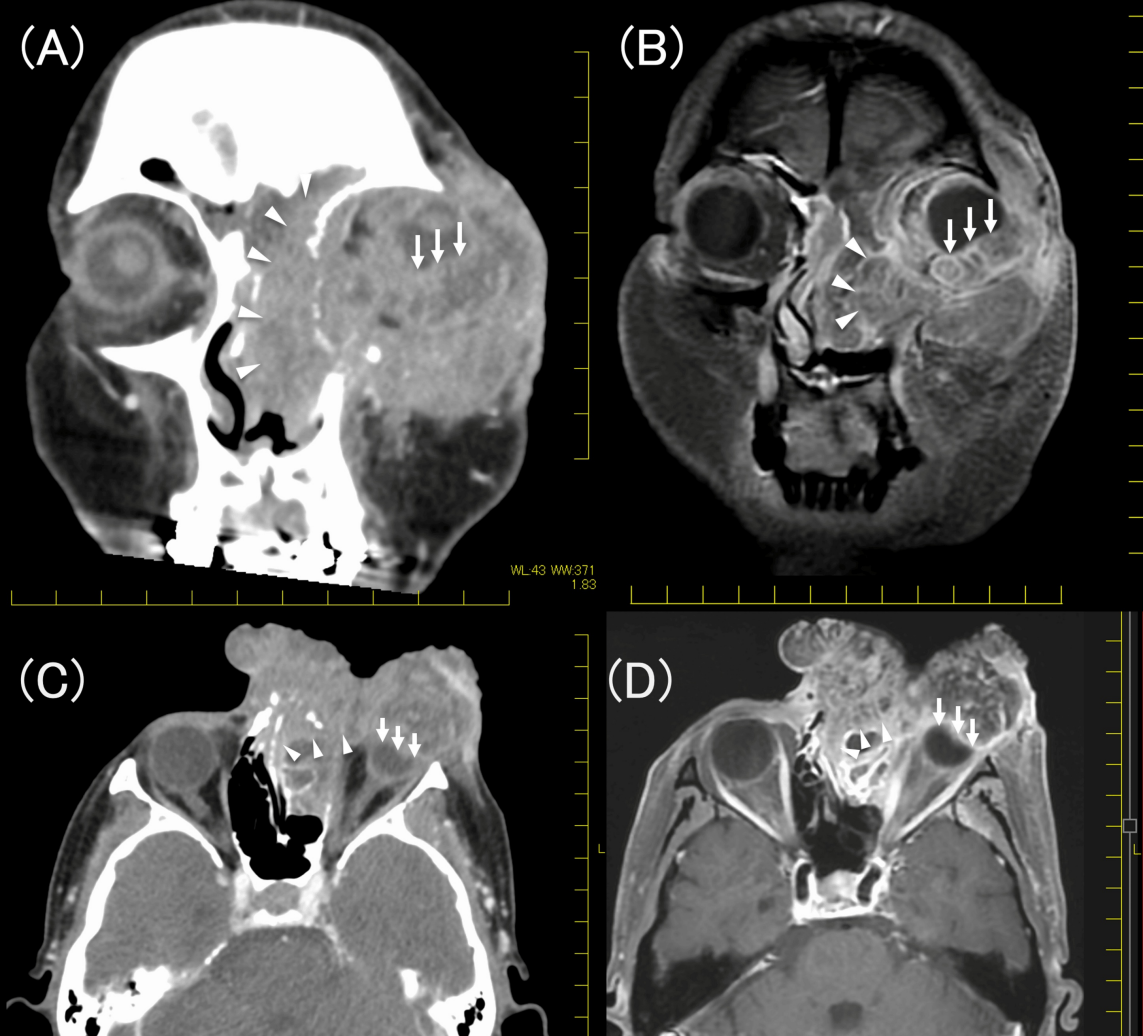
Computed tomography and magnetic resonance images. (A) Coronal contrast-enhanced computed tomography image. (B) Coronal T1-weighted contrast-enhanced magnetic resonance image. (C) Axial contrast-enhanced computed tomography image. (D) Axial T1-weighted contrast-enhanced magnetic resonance image The tumor invaded the orbital contents (white arrows) and sinonasal cavity (white arrowheads)
